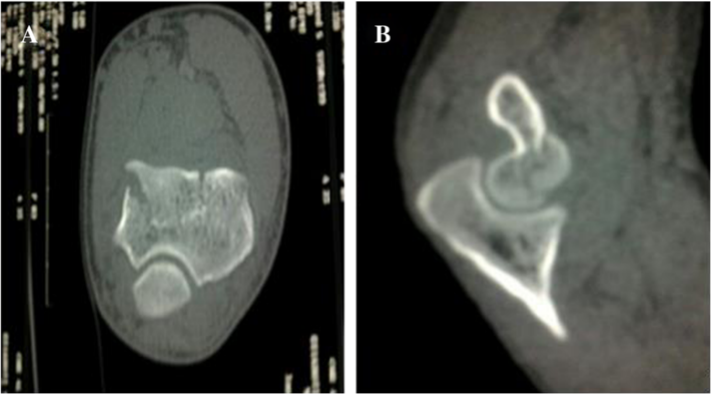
Computed tomography scan. (A) Axial view and (B) sagittal view.
